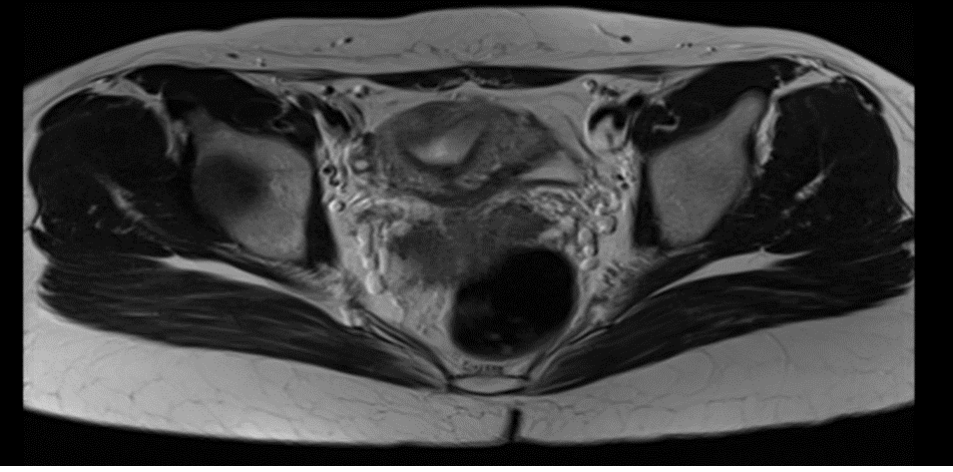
MRI findings two months post hysteroscopic metroplasty confirm complete septum resection and a normal uterine cavity.
